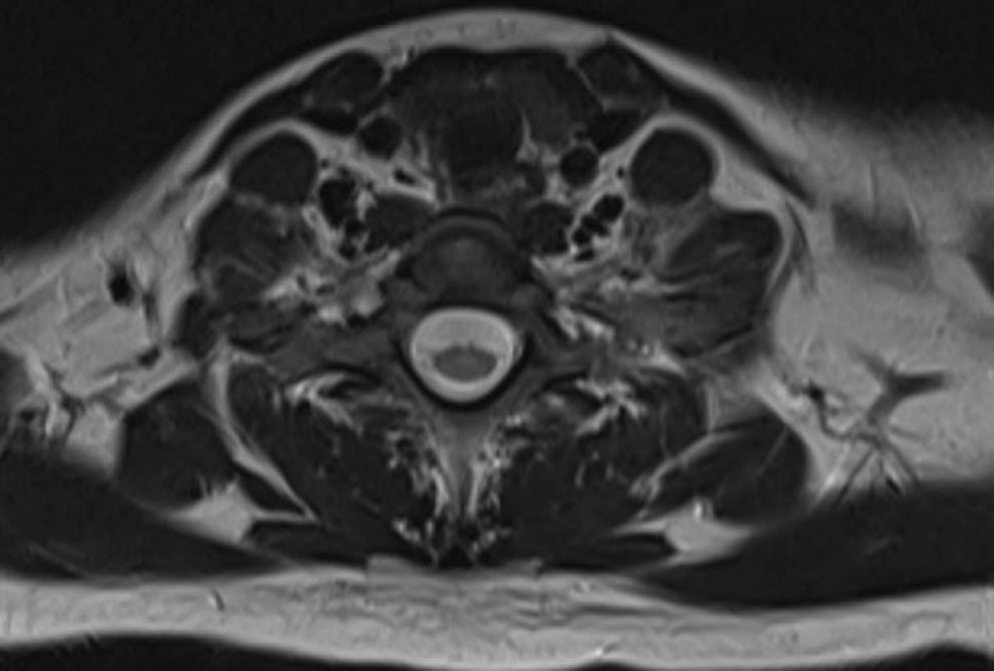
Cervical spine MRI T2‐weighted axial view at C6‐C7 level (normal) MRI, magnetic resonance imaging.
